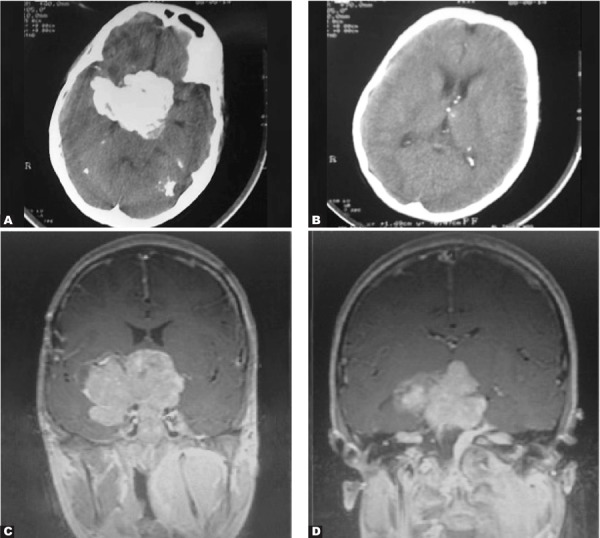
A 16-year-old boy with deafness and skull deformity. A and B. Axial post contrast CT scan C. Coronal post contrast T1-weighted image D.Coronal post Gd injection
